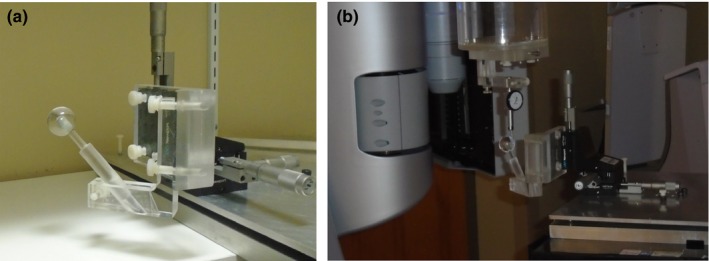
Ball pointer used for couch walkout measurements. (a) The pointer is positioned at cone beam computed tomography isocenter using linear translation stages. (b) A dial gauge mounted on the collimator and in contact with the surface of the pointer measures deviations of the ball as the couch is rotated.
